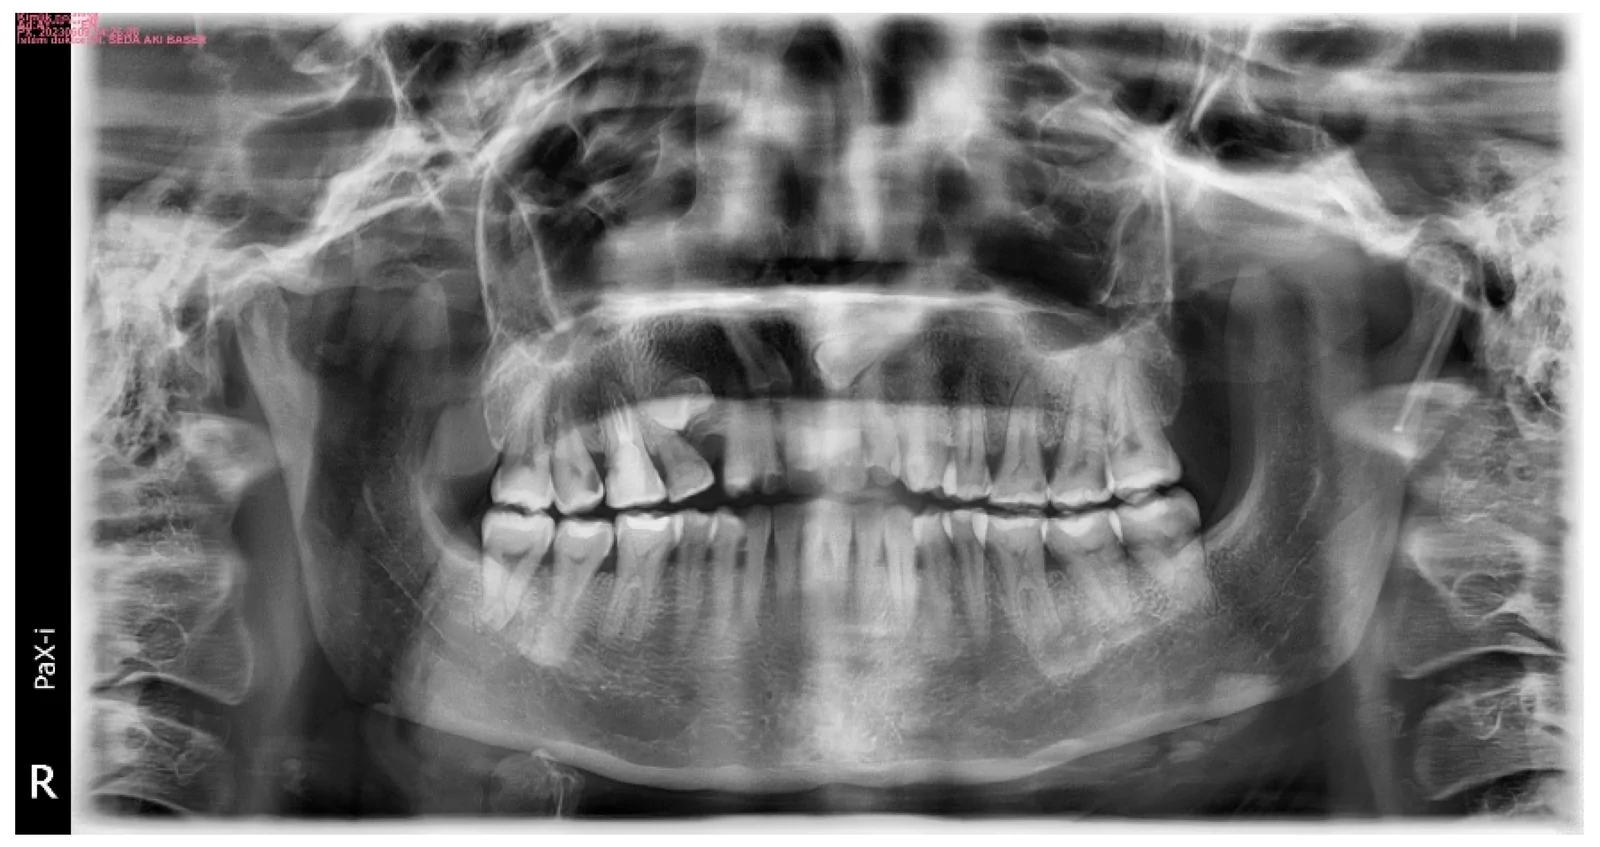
A panoramic radiograph of the father of the patient is shown in Figure 3, demonstrating similar pyramidal molar morphology in the molar teeth.
